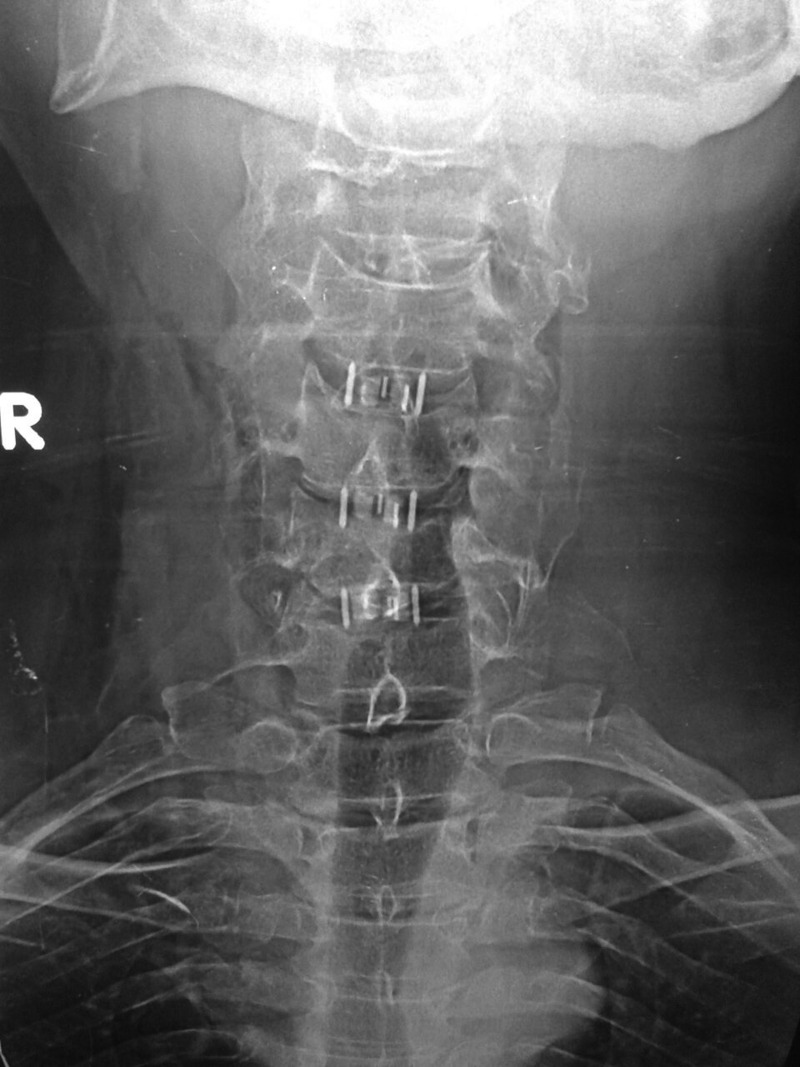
Case Example of a Three-Level ACDF Using Stand-Alone PEEK Cages AP radiograph at three months after surgery demonstrating hardware placement and preservation of disc space heights.
